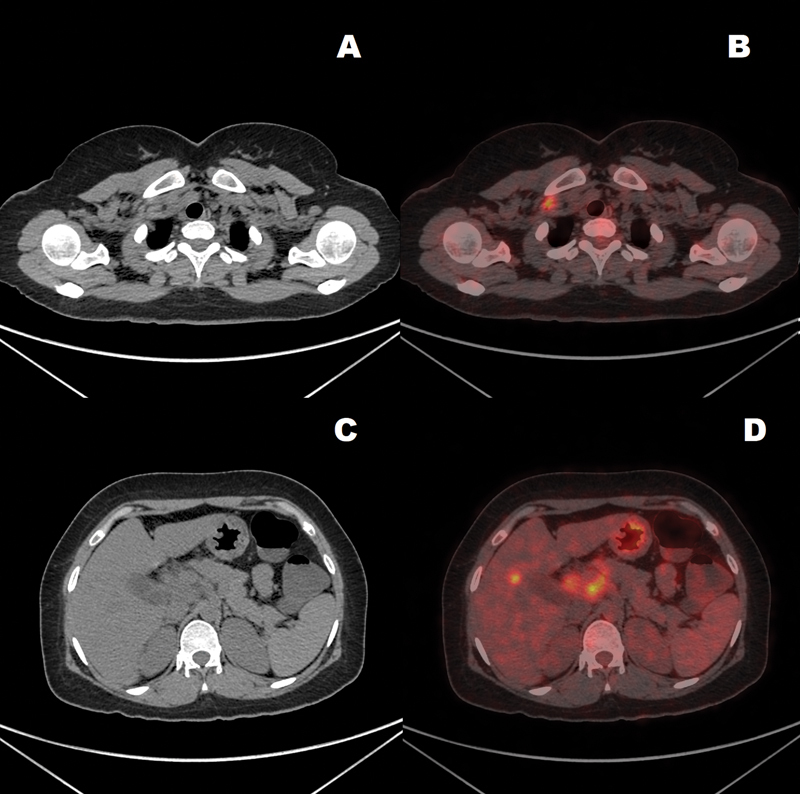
Comparison of CT with corresponding positron emission tomography-computed tomography (PET-CT) images showing additional information provided by the functional imaging. Image panel (
